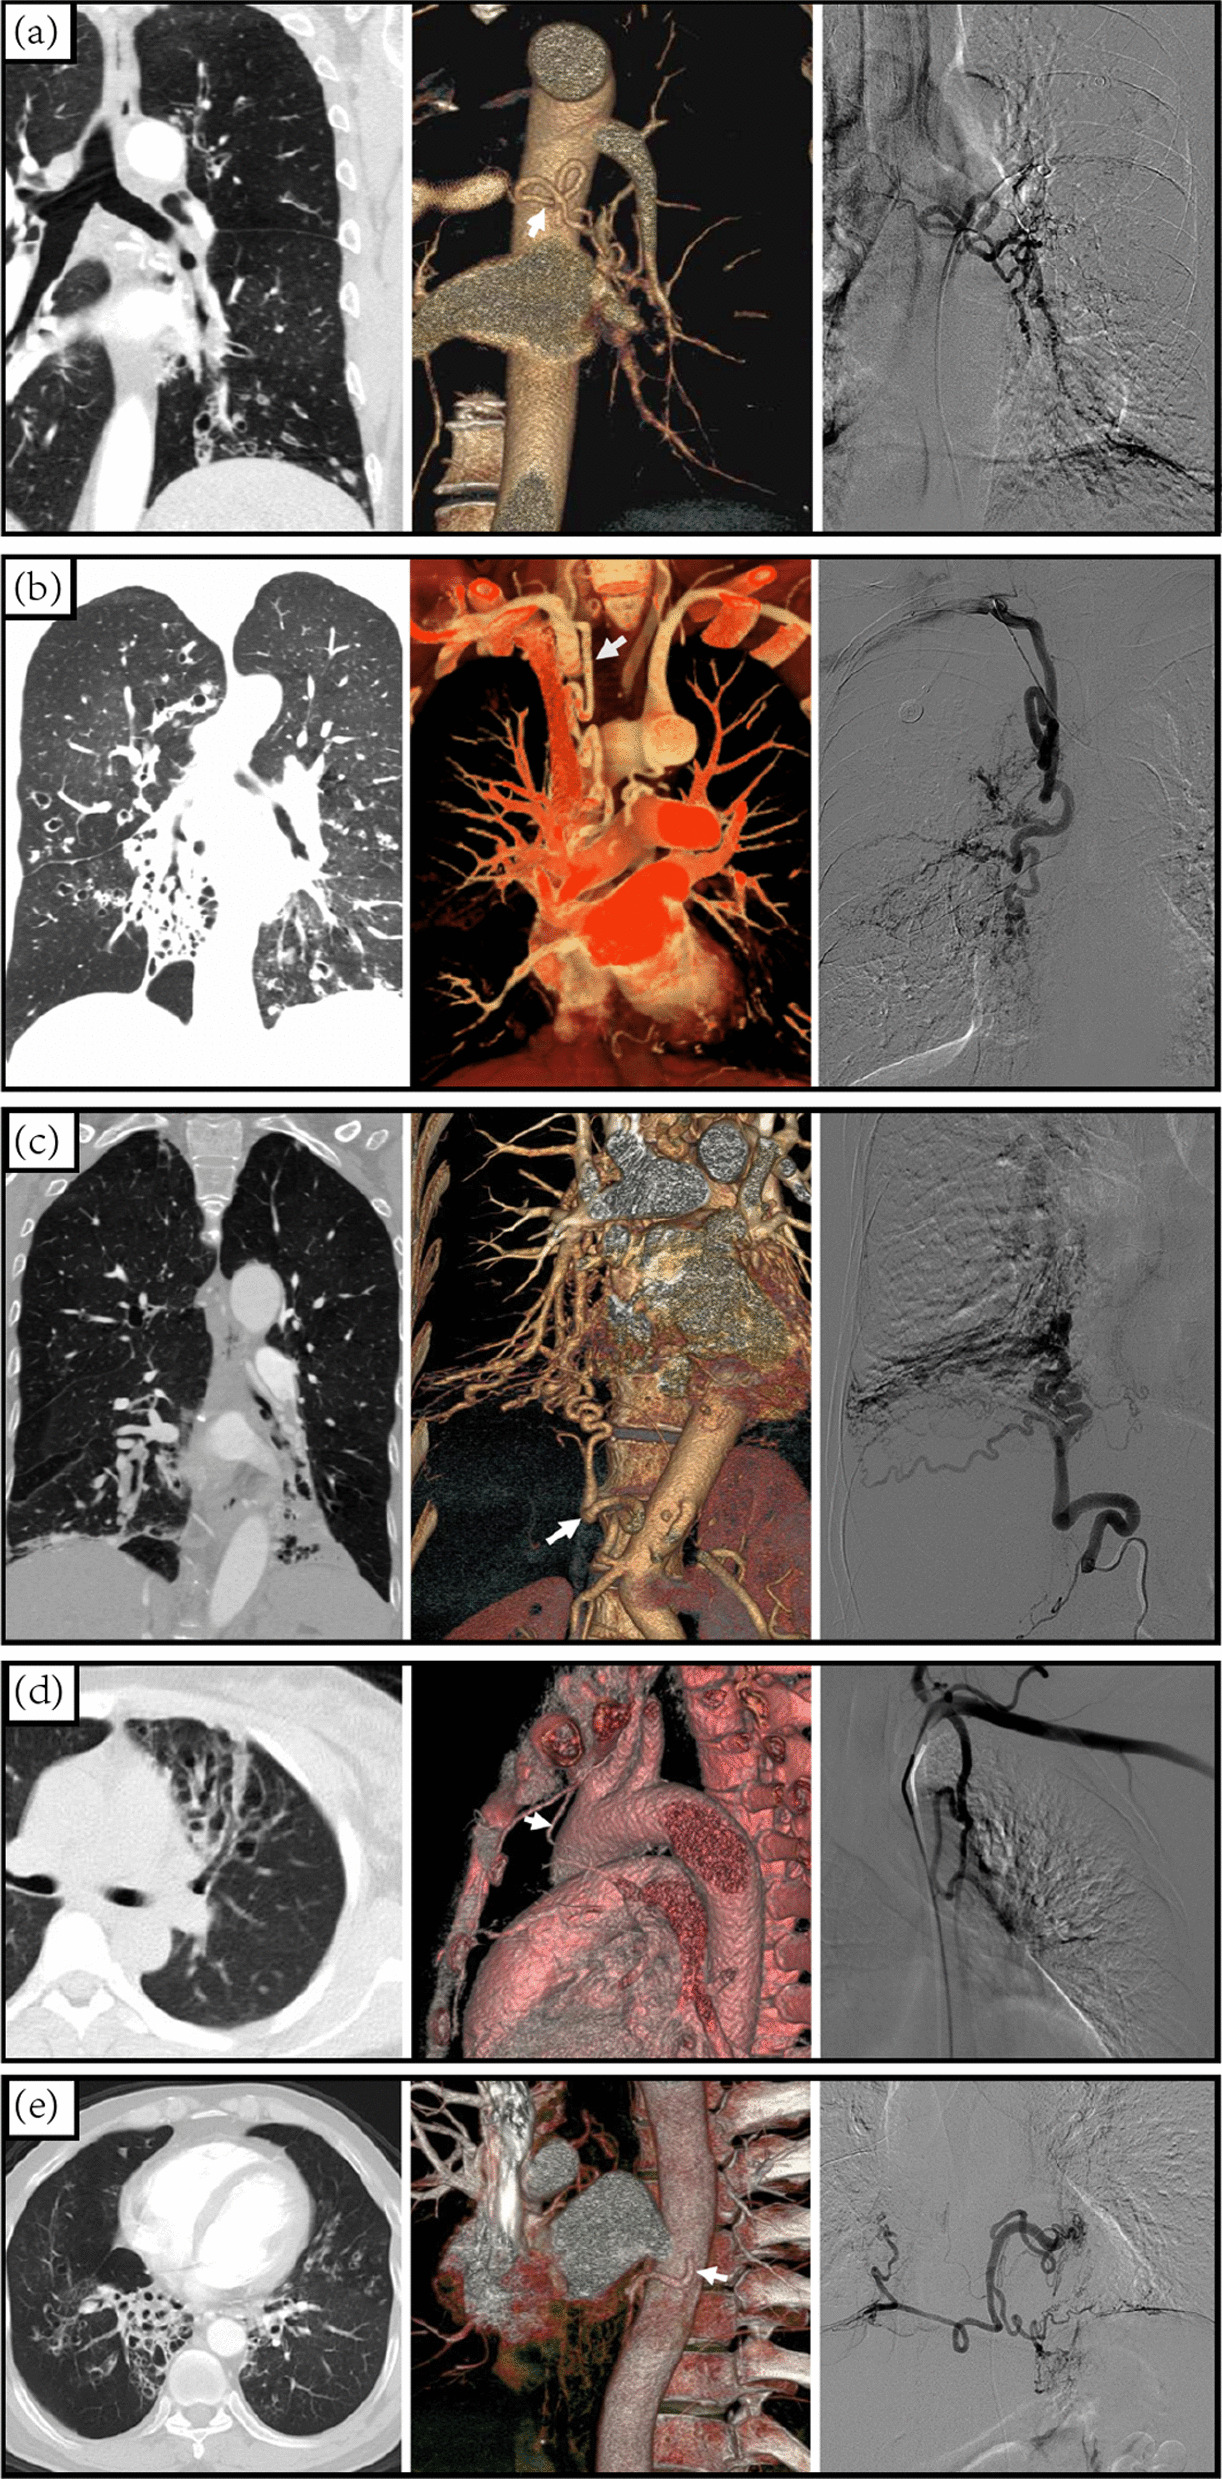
Representative images of BAE for idiopathic bronchiectasis-related hemoptysis with CT (left panel), volume reconstruction (middle panel), and DSA (right panel): (A) Left bronchial arteries. (B) Aberrant bronchial arteries. (C) Right inferior phrenic artery. (D) Left internal thoracic artery. (E) Proper esophageal artery. CT showed bronchiectasis with mucous embolism. The arrow points to the thickening, engorgement, and tortuosity arteries
